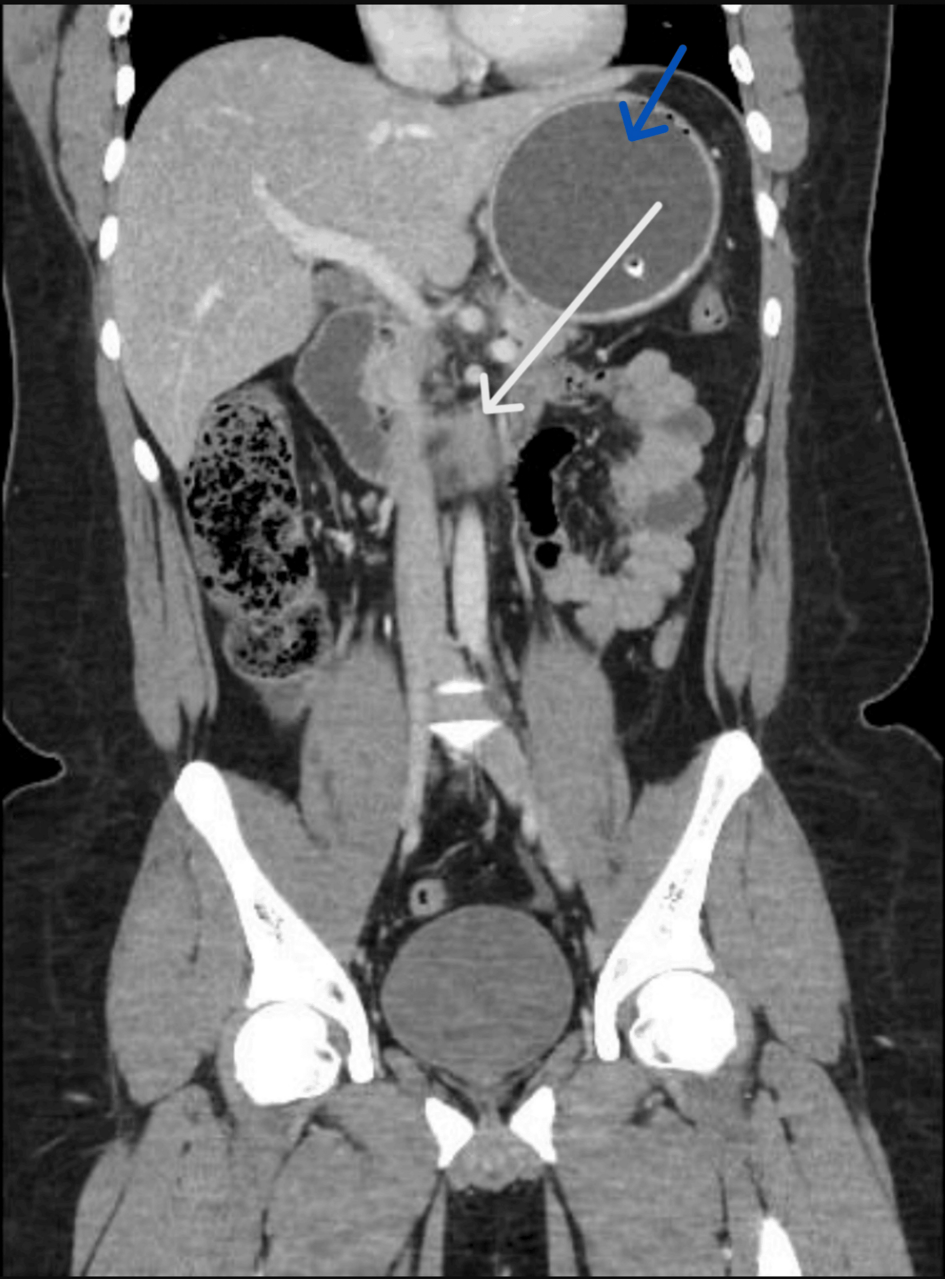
Contrast-enhanced CT of the abdomen showing diffuse enlargement of the pancreas with surrounding peripancreatic fat stranding, consistent with acute interstitial edematous pancreatitis (white arrow). The IGB is visualized in situ (blue arrow) CT: computed tomography, IGB: intragastric balloon
